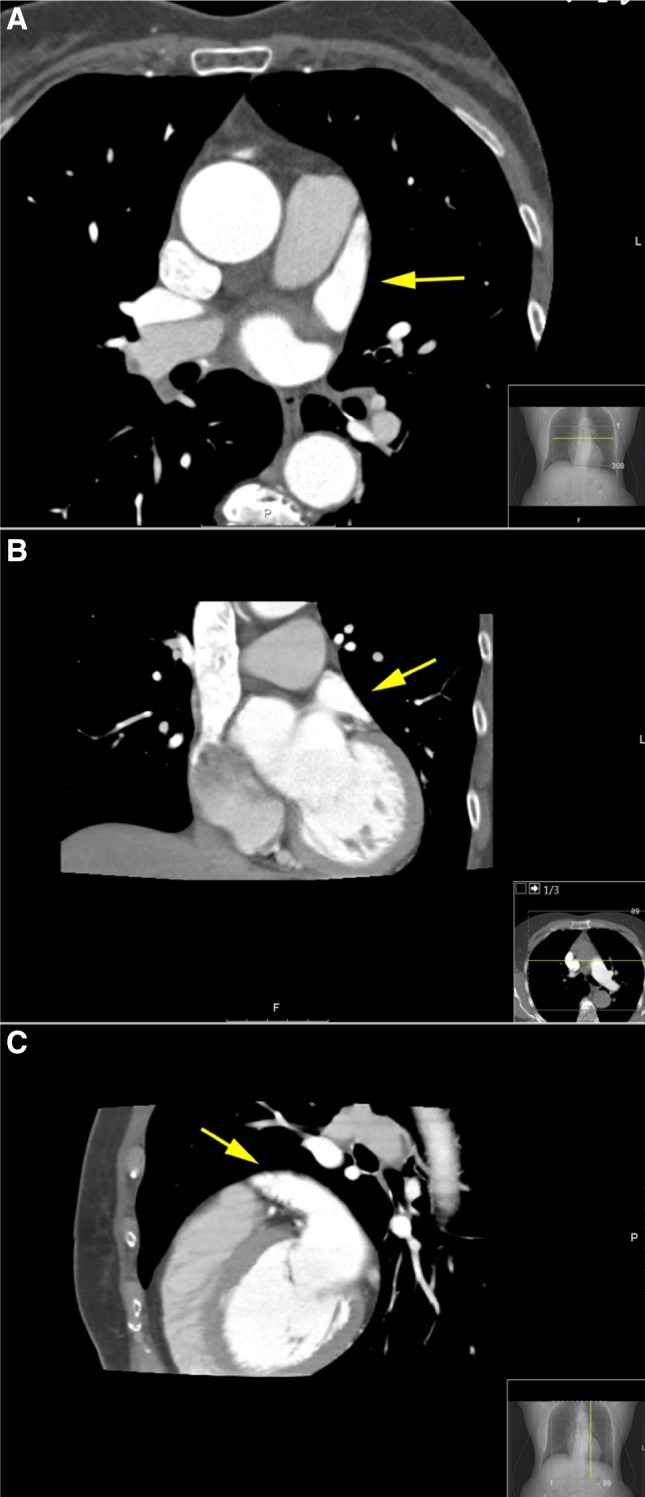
CTA showing complete filling of the LAA (*yellow arrow*), thereby excluding LAA thrombus. a Axial view, b coronal view, c sagittal view
